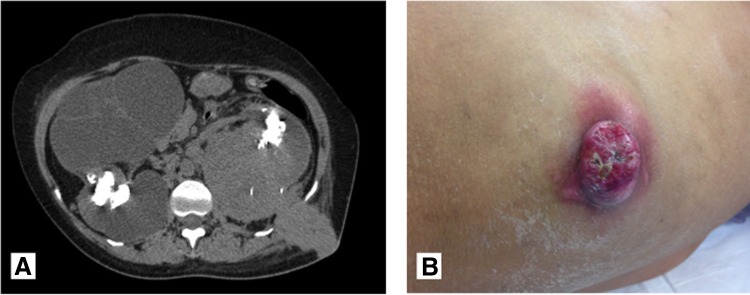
Case 2: Flank mass eruption from the skin at the nephrostomy tube site. (A) CT abdomen pelvis performed 1 month following PCNL demonstrating what was felt to be hematoma extending to the skin. Nonspecific para-aortic lymph node enlargement was also noted. (B) One week following CT scan, the patient developed eruption of a mass-like structure from the nephrostomy tube site. Biopsy revealed well-differentiated keratinizing SCC.
